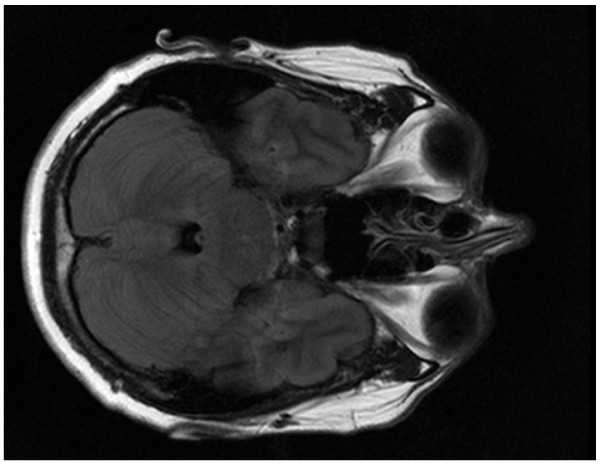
FLAIR seven months after treatment, the lesion completely resolved.
